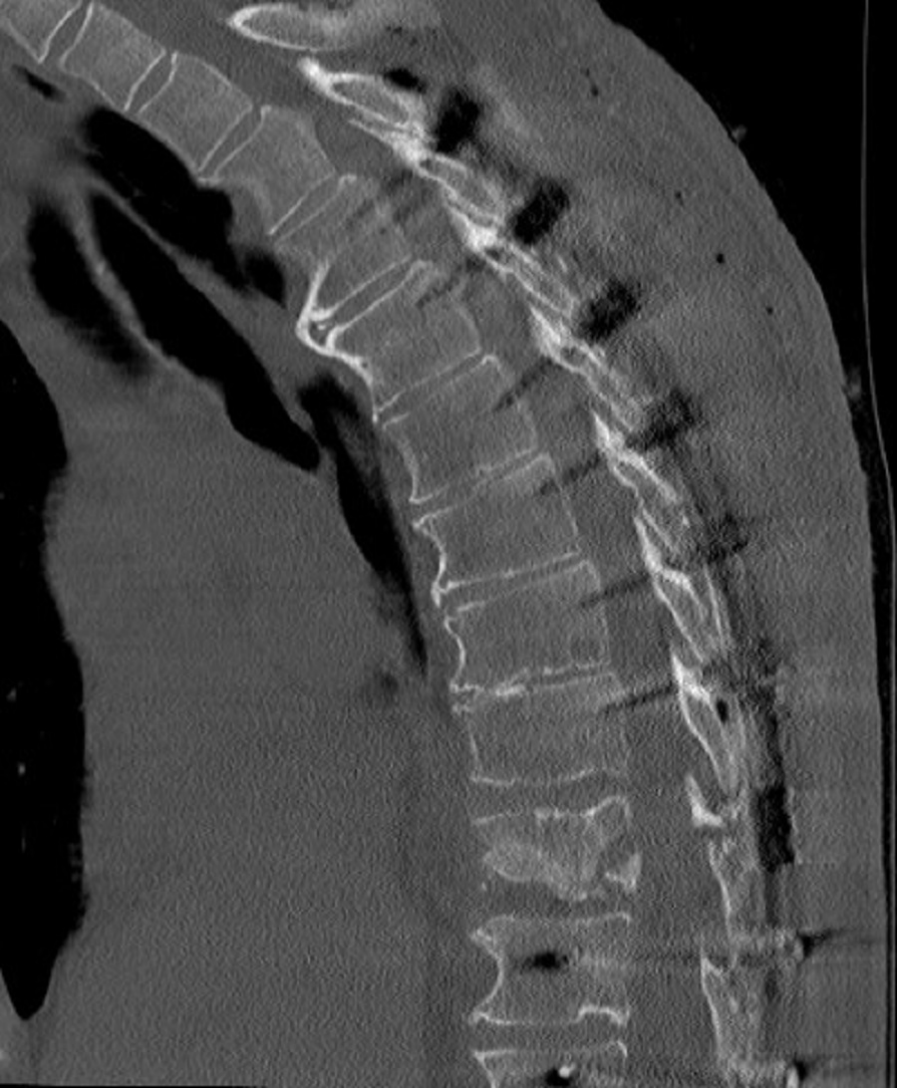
Preoperative sagittal CT demonstrating a T10 burst fracture
